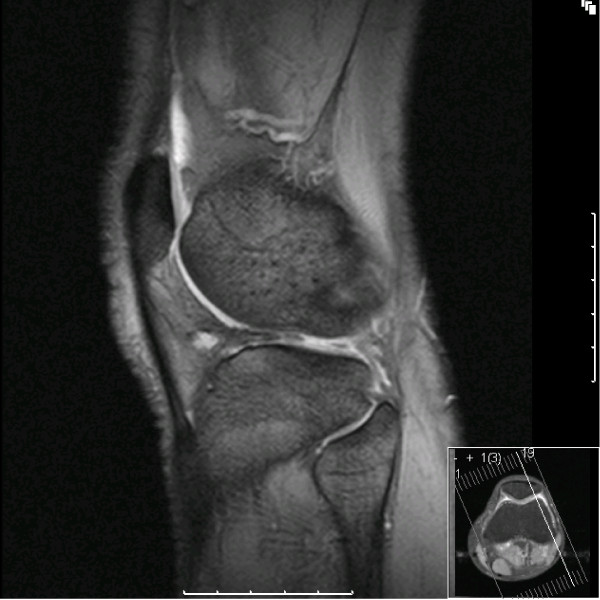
T2 weighted MRI image demonstrating lateral meniscal tear; "Absent bow tie sign".
